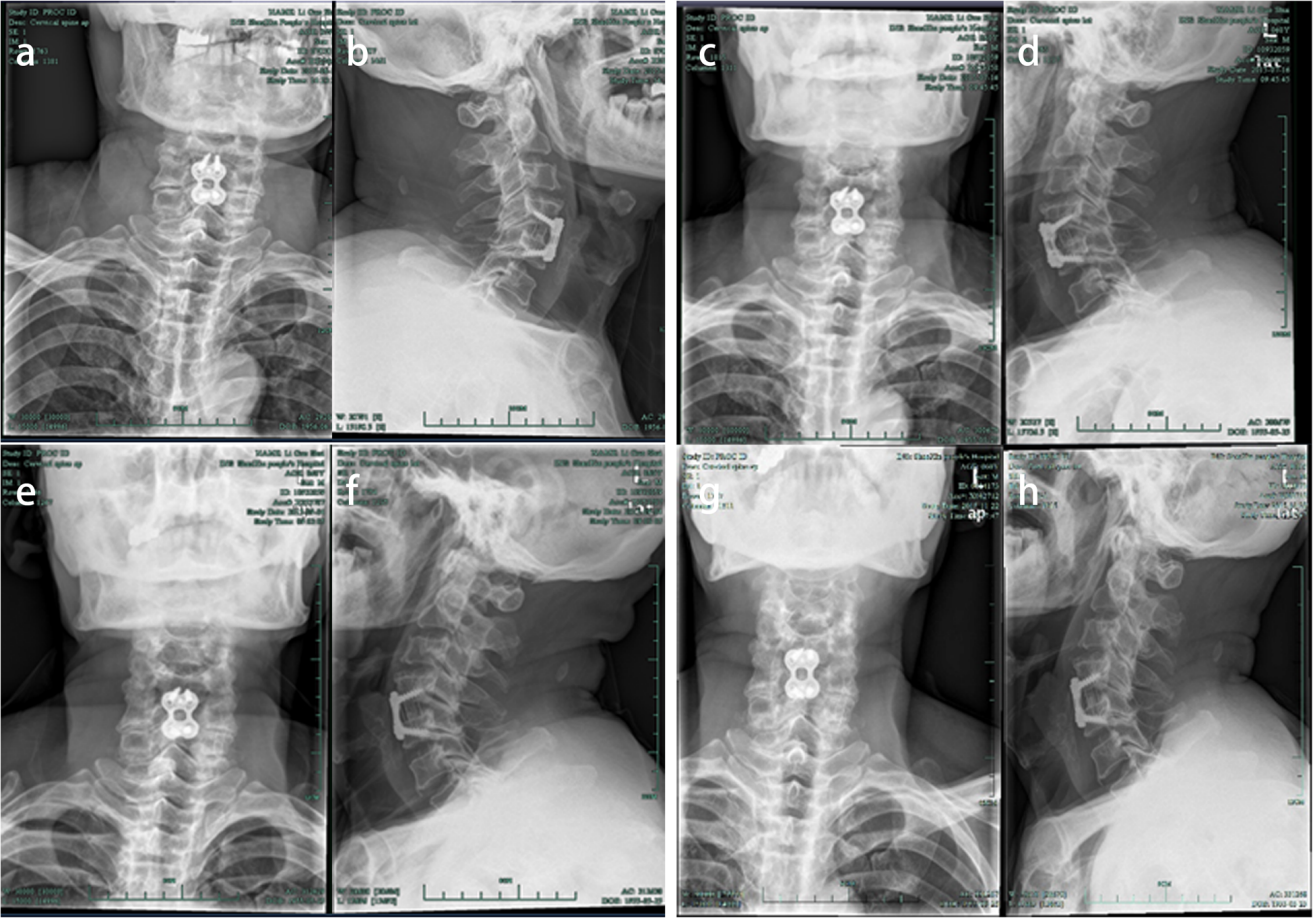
Images of cervical spine radiograph after ACDF showed that the position and length of the plate and screws were satisfactory without looseness or displacement. a, b One day after operation. c, d One month. e, f Three months. g, h Five months
